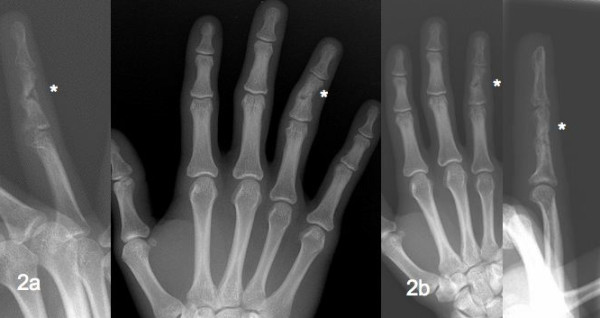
Control X-ray five months after initial operation (a); X-ray six weeks after LIPUS therapy (b).
